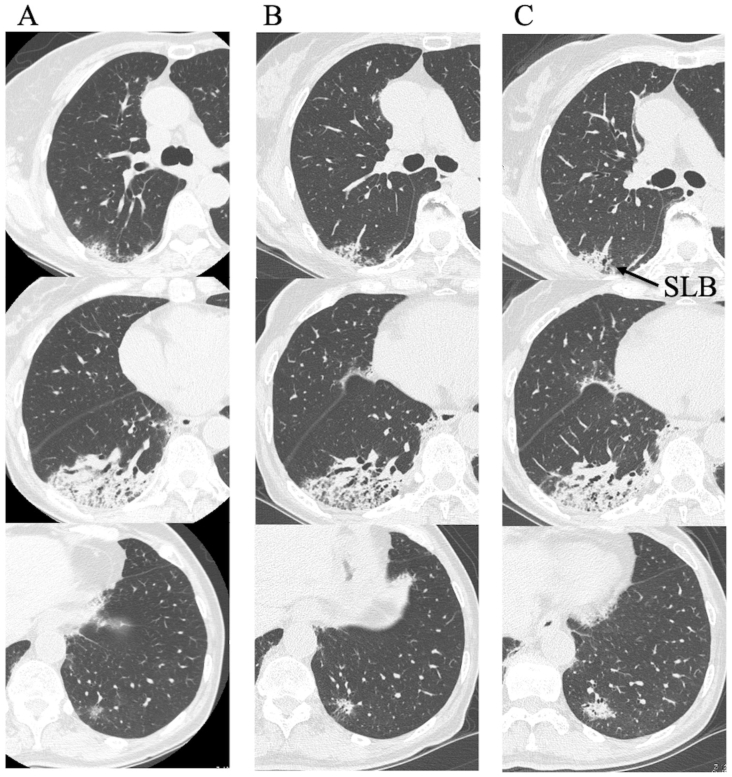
Pulmonary findings of chest high-resolution computed tomography. (A) At the initial visit, subpleural consolidation with volume reduction in the right lower lobe and localized ground-glass opacity in the left lower lobe were present. (B) Two weeks after she began receiving prednisolone (PSL), consolidation had not improved. (C) Two months after discontinuation of PSL, bilateral consolidation developed. Then, a surgical lung biopsy (SLB) of the right upper lobe was performed (arrow).
